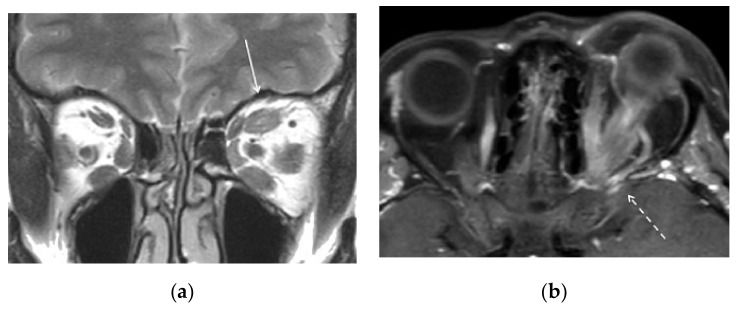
(a) MR, T2-weighted image, coronal plane, confirming a bilateral global hypertrophy of the extrinsic muscles, more pronounced to the left superior rectus (white arrow). (b) MR, T1-weighted fat-sat postgadolinium image, axial, showing a slightly enlarged left superior ophthalmic vein (dashed arrow), partially pinched by the superior rectus muscle.
